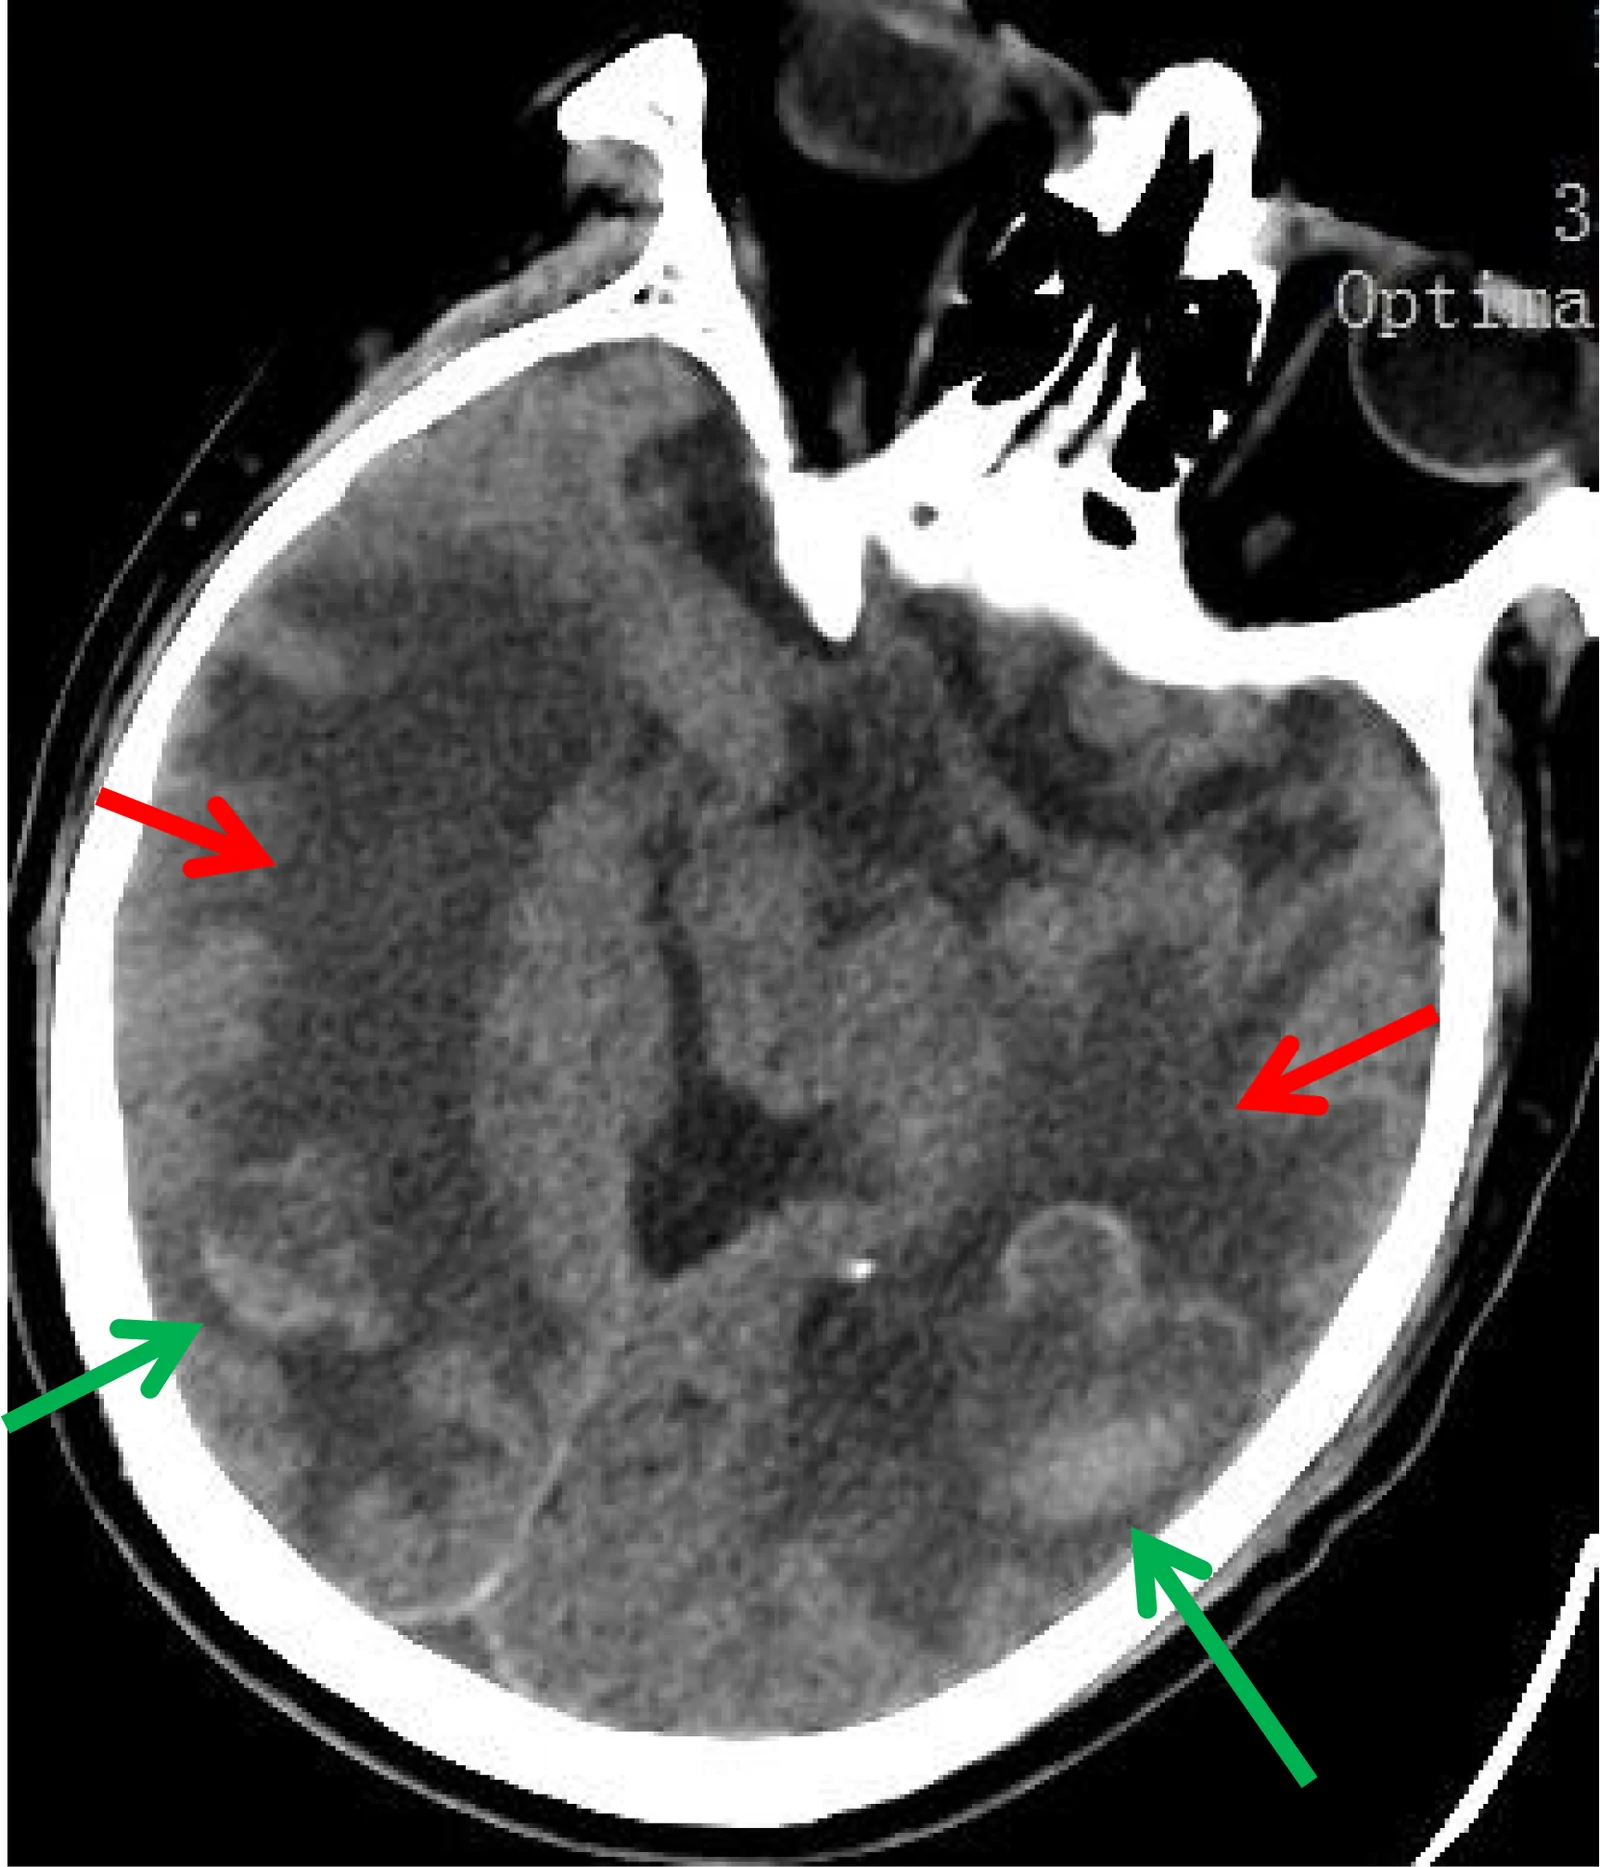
Head computed tomography image. Multiple cystic and solid lesions were present in both cerebral hemispheres, with local hemorrhage (green arrow) and extensive edema (red arrow).
